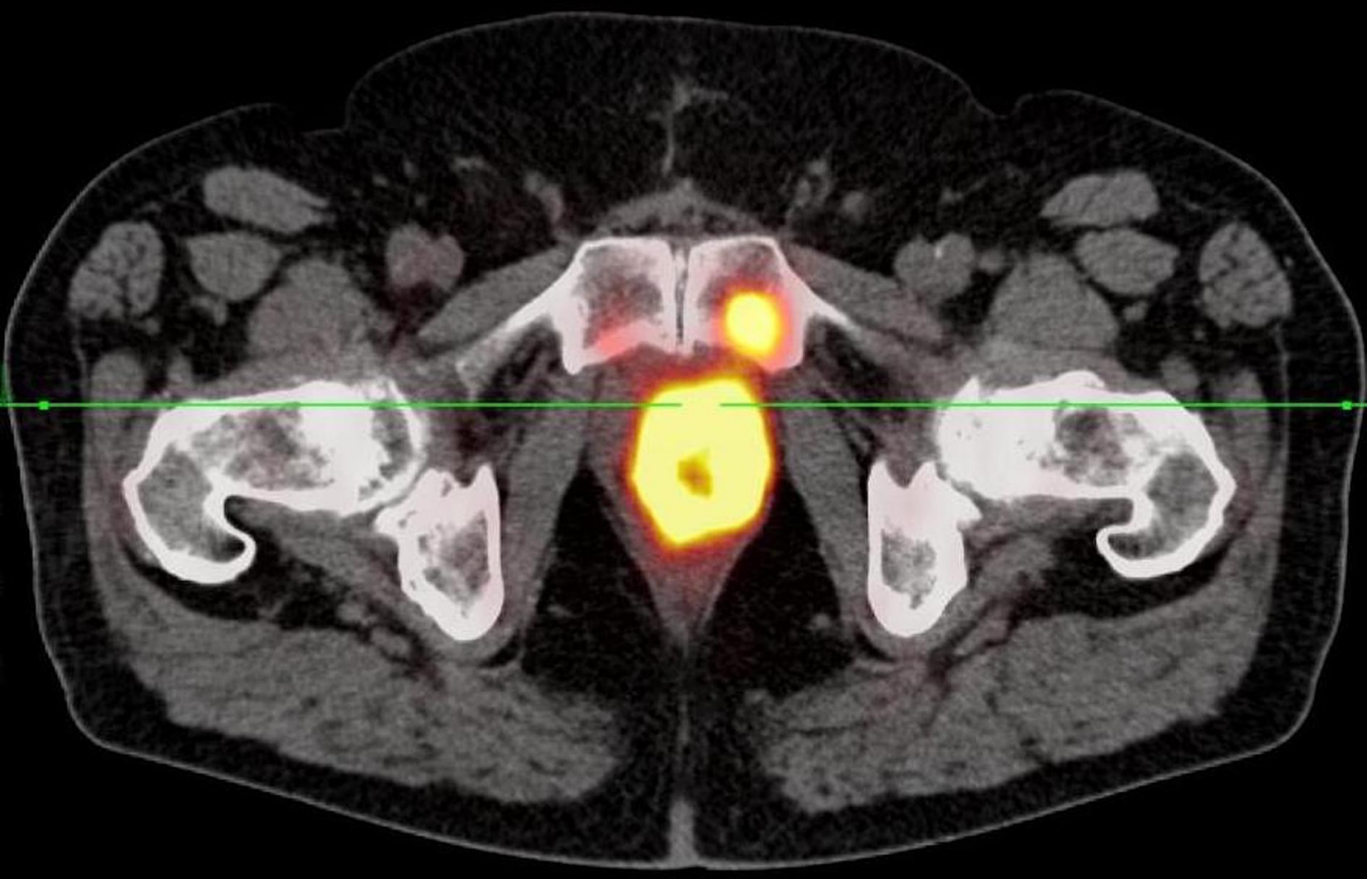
68Ga-PSMA-11 PET/CT shows a bone metastasis in the symphyseal os pubis on the left.
